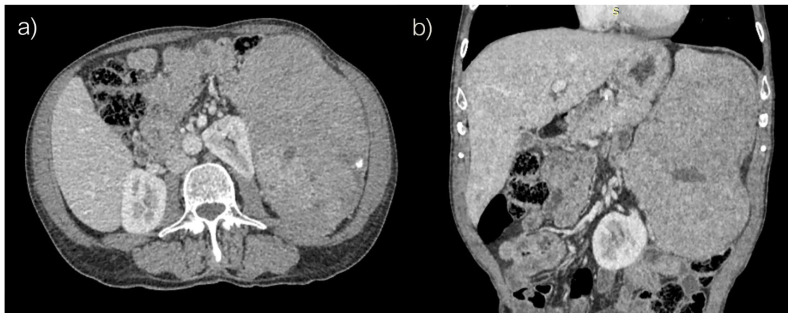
Abdominal computed tomography, (a) axial and (b) coronar scans.
